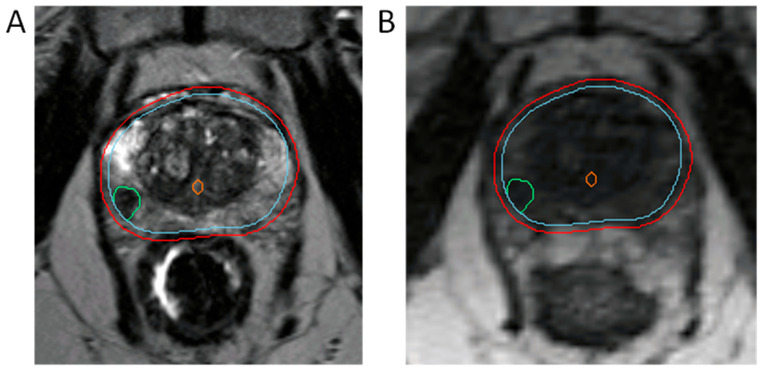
MR-Linac-based integration of simultaneous integrated boosting of the dominant intraprostatic lesion (DIL). (A) T2-weighted diagnostic MRI. (B) MR-based planning image on the MRIdian device. DIL is in green, urethra is in orange, CTV is in cyan, 2 mm isotropic PTV expansion is in red.
